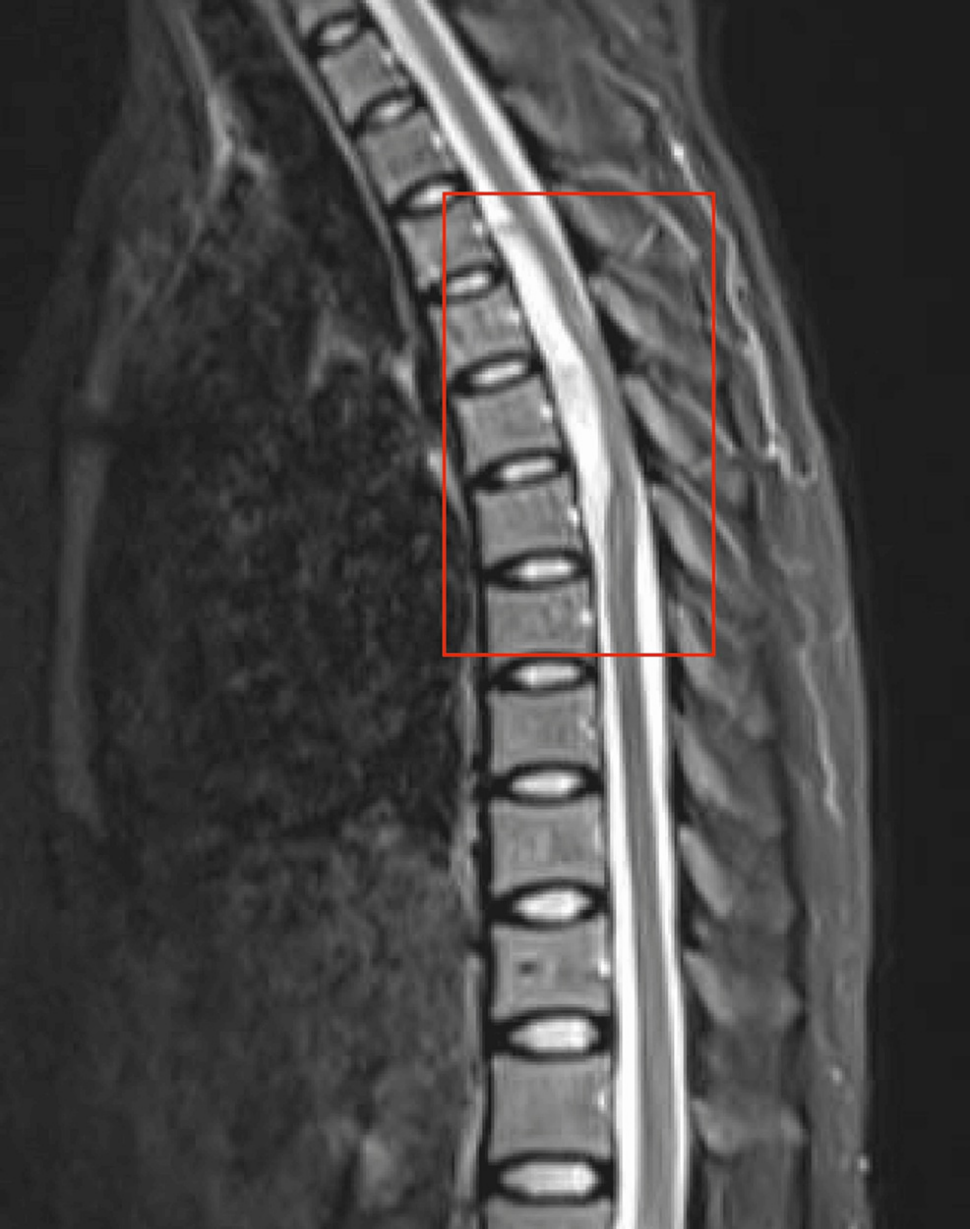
T2-weighted MRI of the thoracic spine portrays the epidural hematoma that ranged from T2 to T8.
